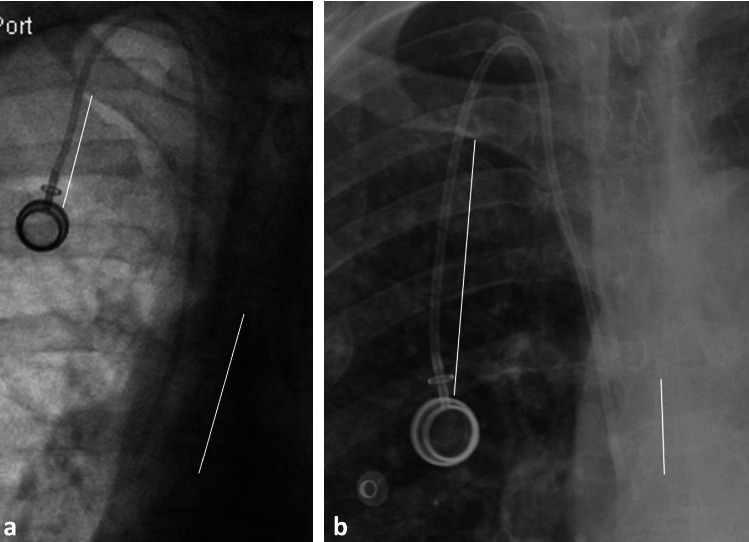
Right-sided port measurements. (a) Magnified fluoroscopic image of initial right-sided port insertion demonstrating typical measurement locations (solid white lines). (b) Magnified follow-up chest radiograph demonstrating typical measurement locations (solid white lines). This example is a 66-year-old female with a right-sided chest port in situ and a 2-month interval between imaging. Δ catheter tip-to-carina: −19 mm (cephalad, retracted); Δ port: +36 mm (caudal, protracted); zone on fluoroscopy: distal superior vena cava (Zone A); zone on follow-up: midsuperior vena cava (Zone A).
